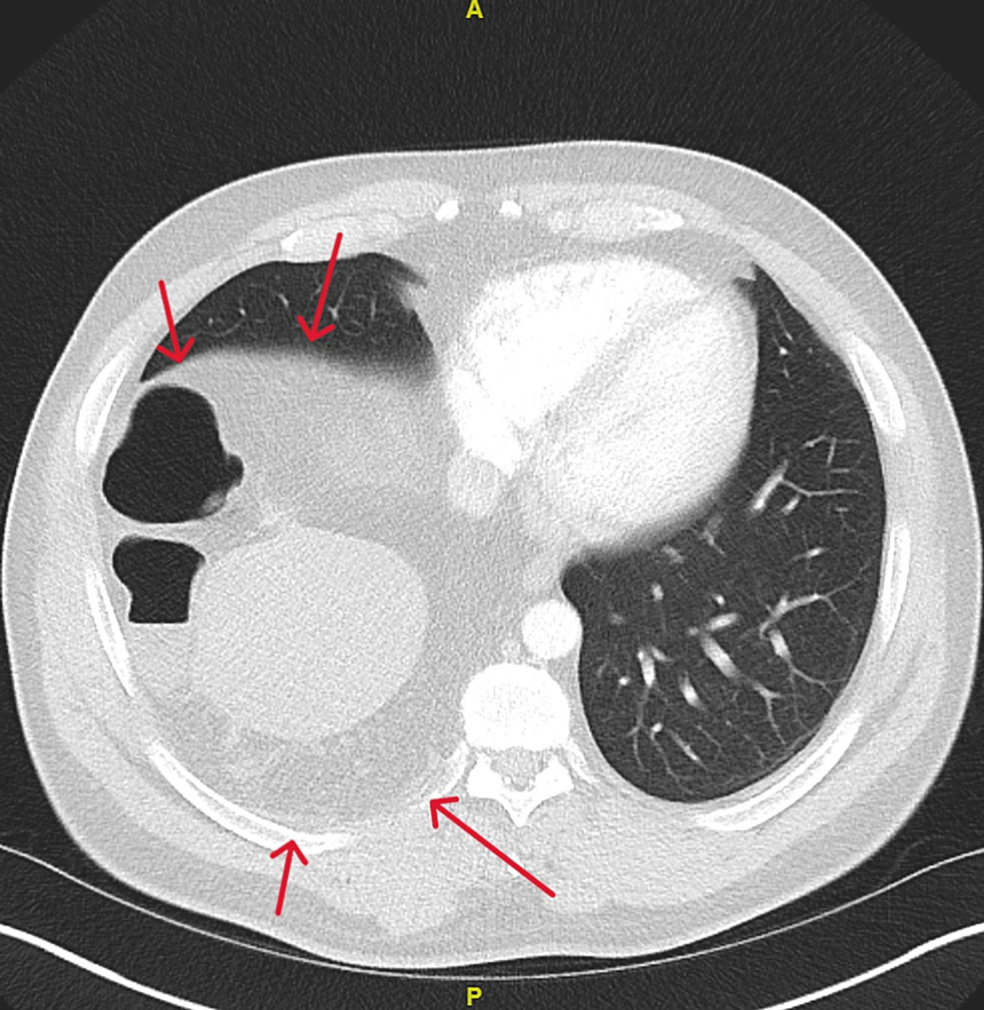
Chest CT showing liver and large bowel within the right lung field. Red Arrows: Large bowel
